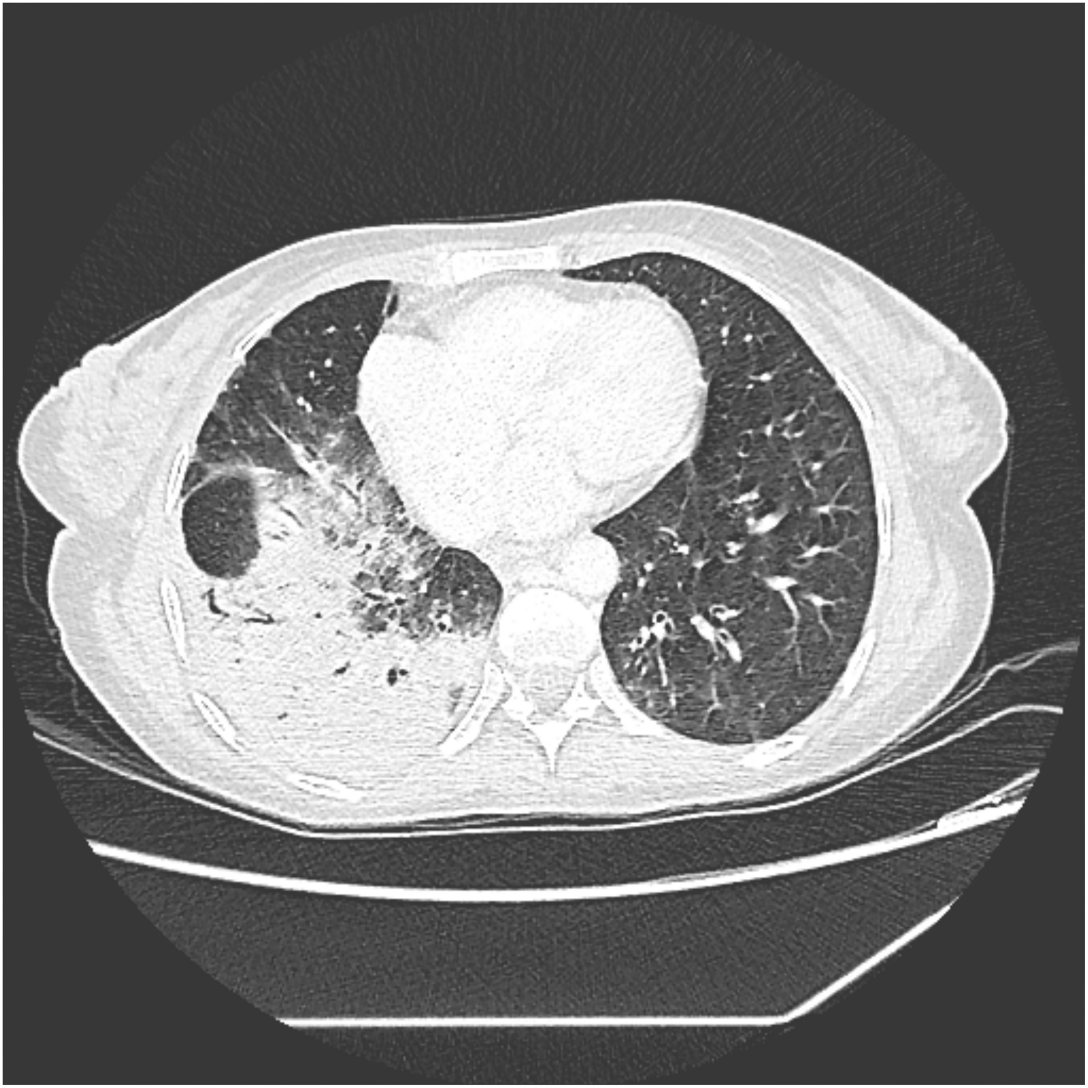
Slice of CT scan showing parenchymal consolidation of the right lower lobe.
